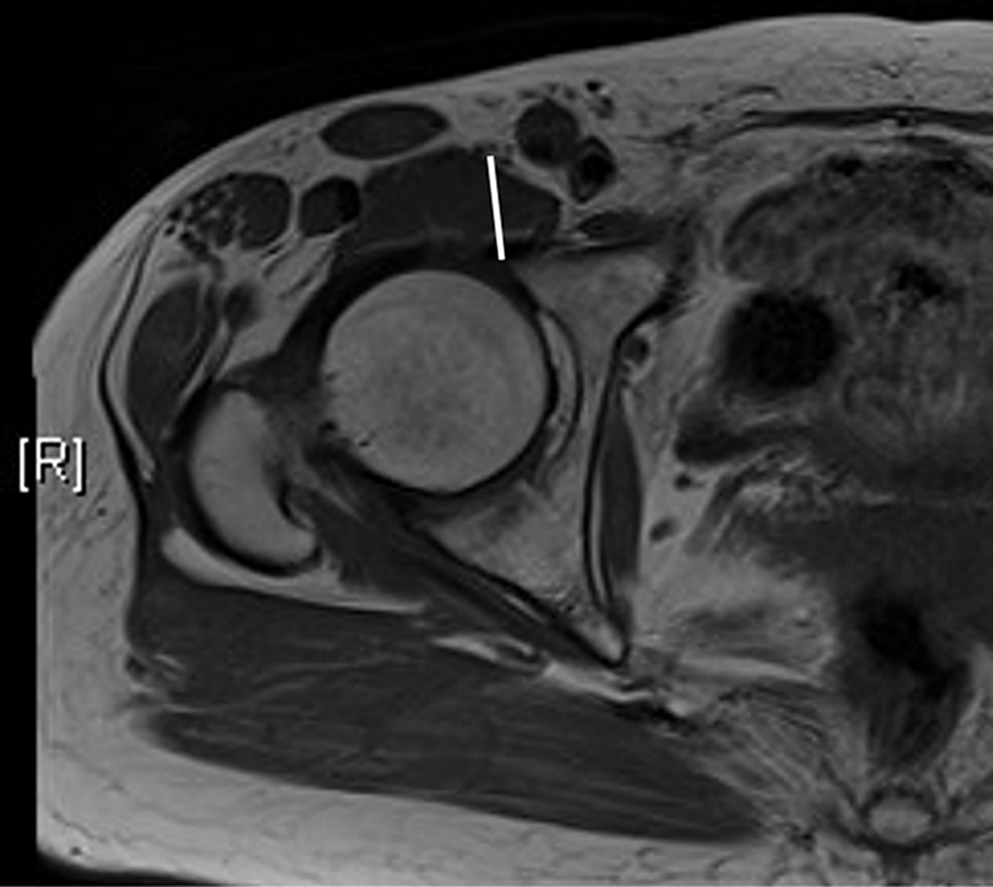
MRI measurement of the Dfn. Axial T1–weighted MRI scan of the right hip at the level of the center of the femoral head in a 67-year-old woman. MRI, magnetic resonance imaging; white line: dFN. dFN, distance between the anterior acetabular edge and femoral nerve
